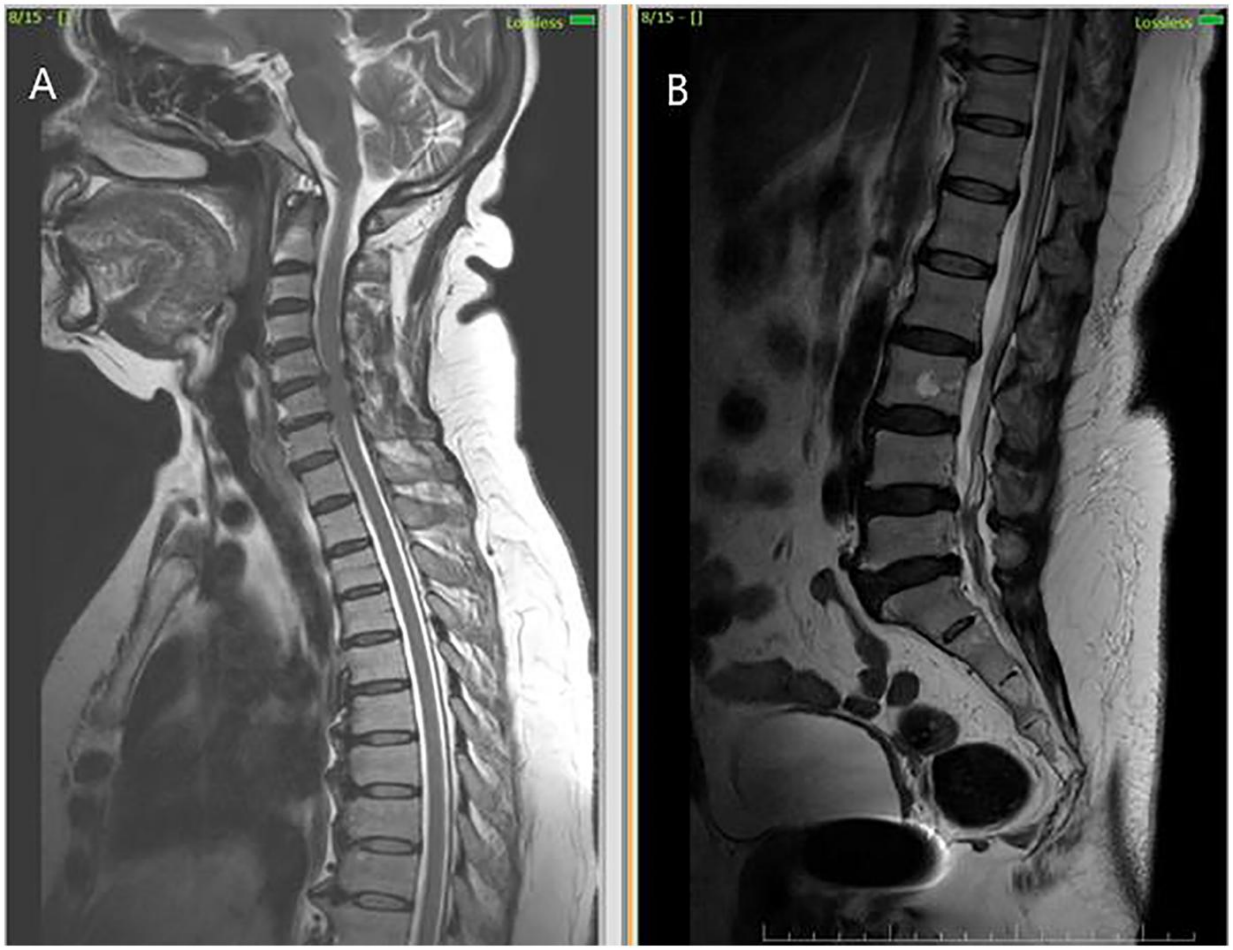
MRI cervical and lumbar spine findings suggestive of spondylosis. (A) Sagittal T2-weighted MRI shows cervical spondylosis with disc degenerative changes (B) Sagittal T2-weighted MRI shows lumbar spondylosis with disc degenerative changes.
